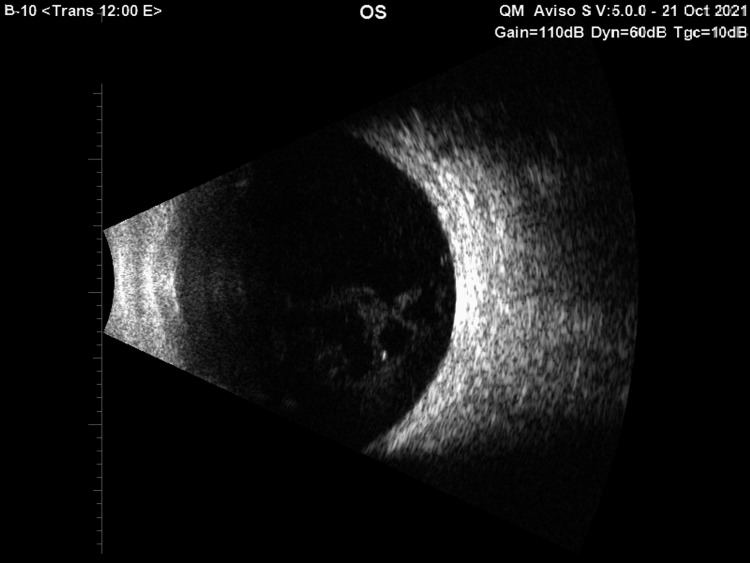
A bright scan (B-scan) ultrasound image showing mild vitreous opacities with cavitations and no significant retinal or choroidal thickening
